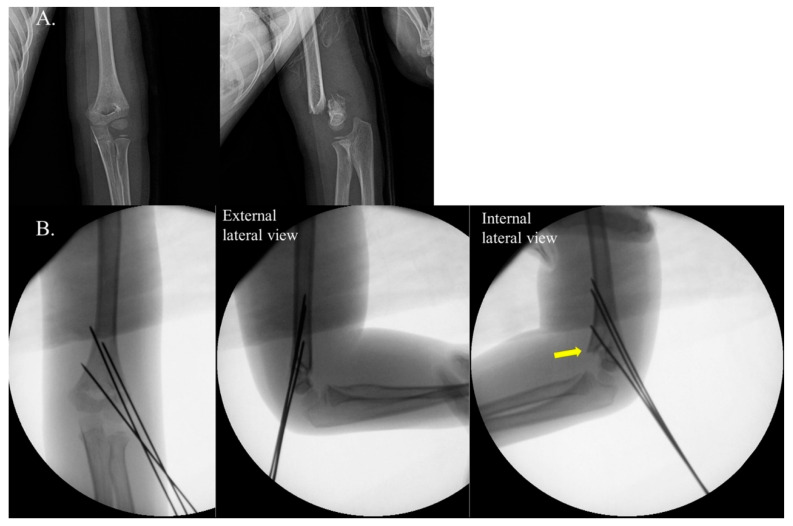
Example of positive internal rotational stress test (IRST) results in a 5-year-old boy with a Gartland type III supracondylar fracture. Panel (A) shows the preoperative radiographs. Panel (B) depicts C-arm images demonstrating a positive IRST. Good bone contact and well-maintained reduction are observed in both the anteroposterior (AP) and external lateral views. However, note (yellow arrow) the loss of reduction due to fragment rotation in the internal stress lateral view.
